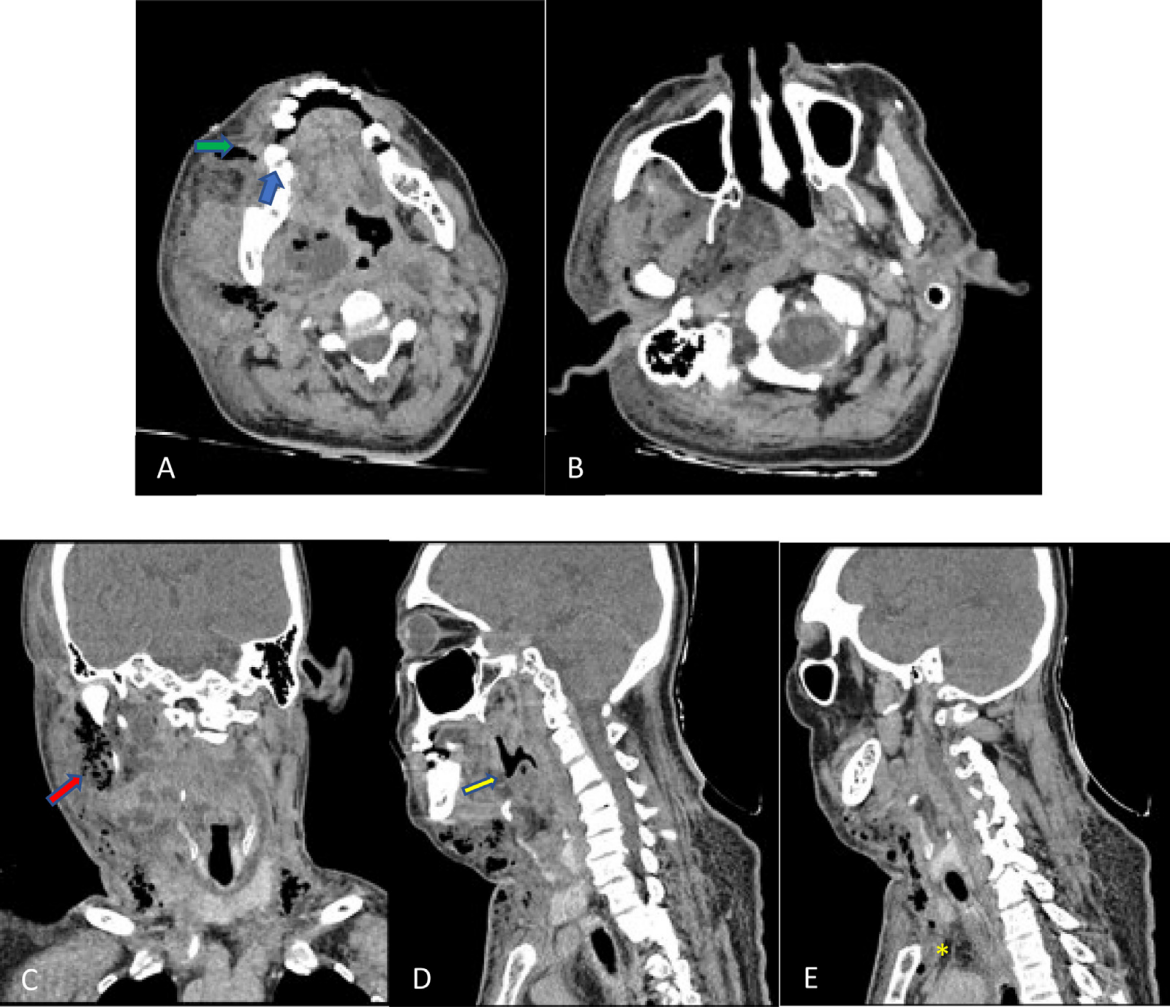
A contrast CT scan of the neck's soft tissue. (A) Periapical abscess of the right first molar (green arrow, axial image) with soft tissue swelling around it that extended to the right sublingual space (blue arrow, axial image), (B) The abscess extended to the buccal space, cutaneous-subcutaneous of temporomandibular, right and left parapharyngeal space, and danger space. (C) Right stylomandibular canal (red arrow, coronal image) (D) The abscess caused airway obstruction with a diameter ±2 mm (yellow arrow, sagittal image) (E) The abscess also extended to mediastinal caused mediastinitis (yellow asterix, sagittal image).
